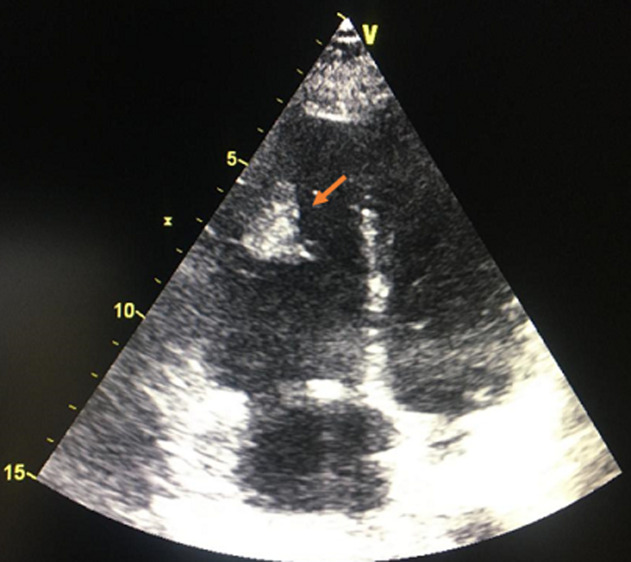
echocardiography - an apical four chamber view showing the presence of a mass on the lateral wall of the right ventricle (arrow)
